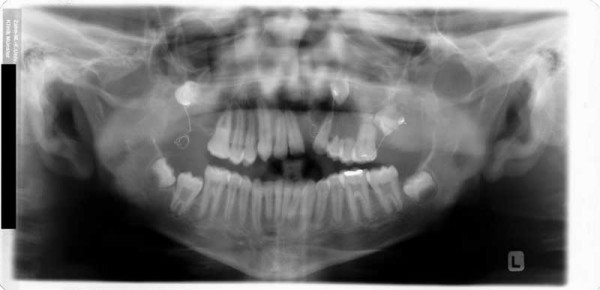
Age 14.3. Panographic x-ray. Situation after extraction of 21 due to internal resorption at age 13.5. Situation after surgical removal of 18 and 28 and surgical exposure and bonding of 17, 23, and 27; before attempting orthodontic extrusion of 17, 23, and 27. Note severe idiopathic resorption of teeth 24 and 25.
